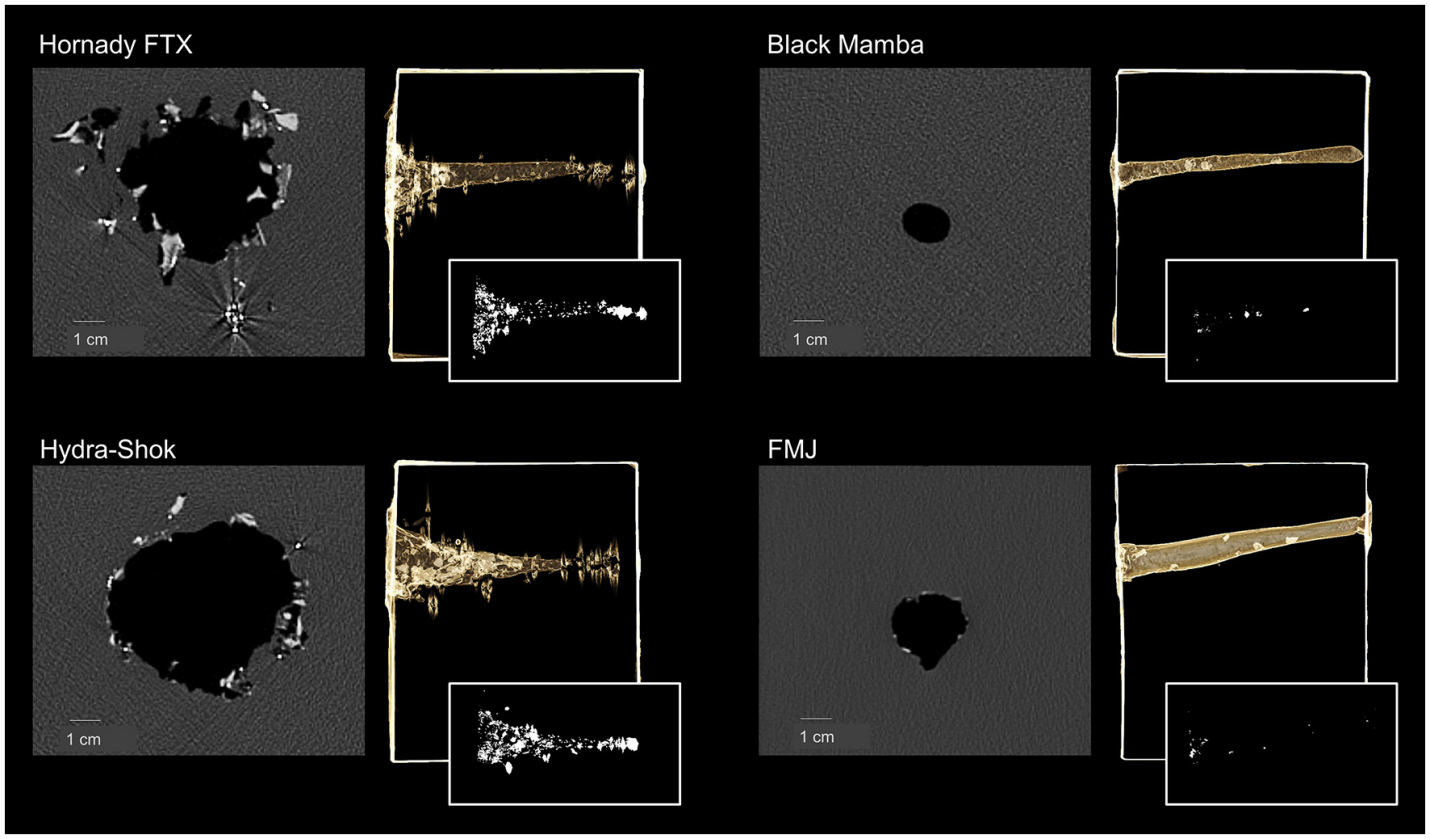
Transverse cross-sectional CT image through the cavity immediately after the perforation of the frontal bone sample and penetration into the soap block (for each bullet type, the left image in grayscale), volume rendering to show the entire cave in the soap block (for each bullet type, the right image kept in the color of the soap block), and maximum intensity projection to highlight bullet fragments along the cavity (for each bullet type, the right small image in black and white).
